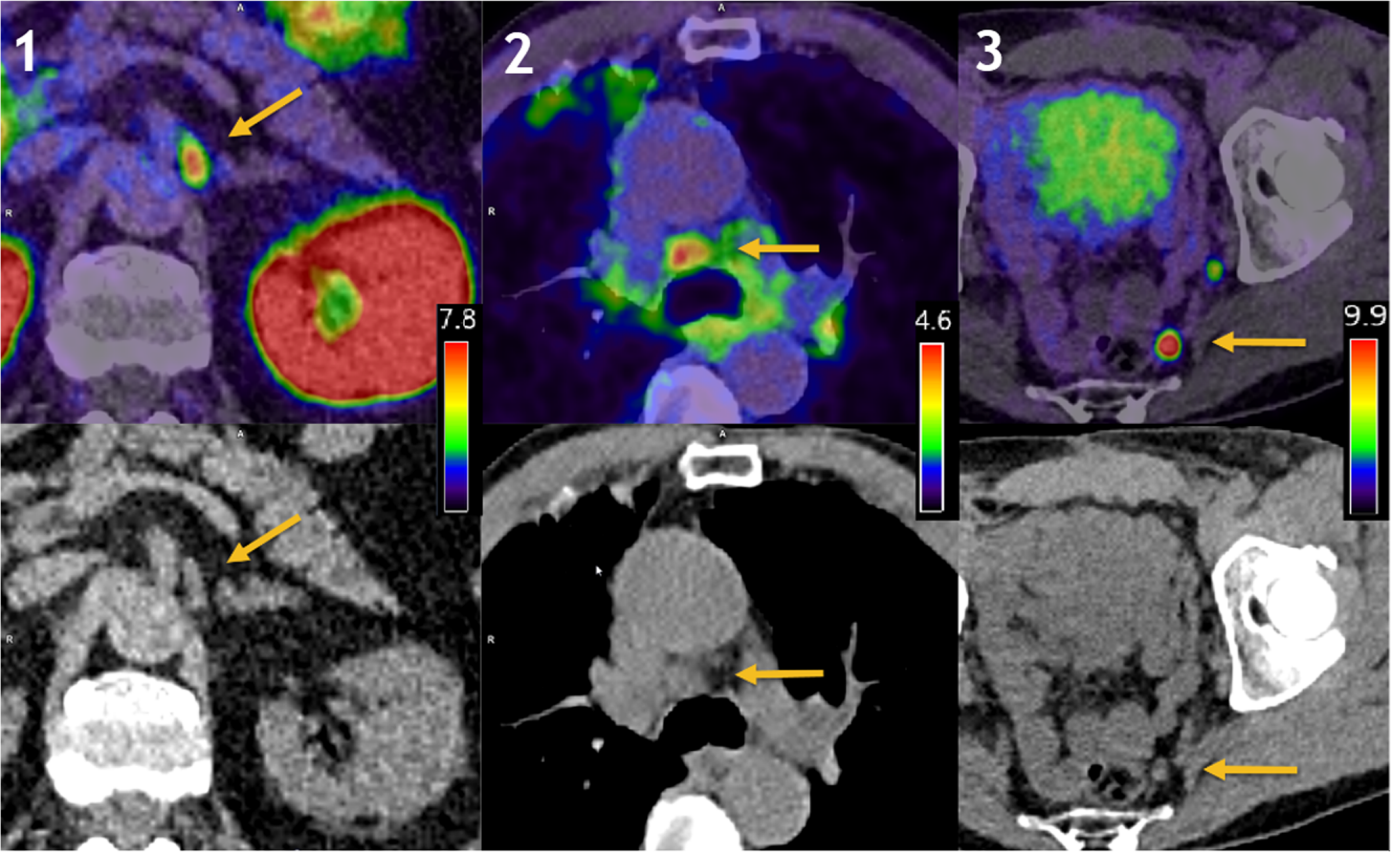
Example images showing a benign ganglion with intensive PSMA-uptake (1, leftmost), equivocal uptake in a mediastinal lymph node (2, middle), and clearly pathological uptake (3, rightmost) in a pelvic lymph node. Top row fusion of PET and CT, bottom row. The appropriate window levels affording the best appreciation of the lesion are shown in the legend for each panel
